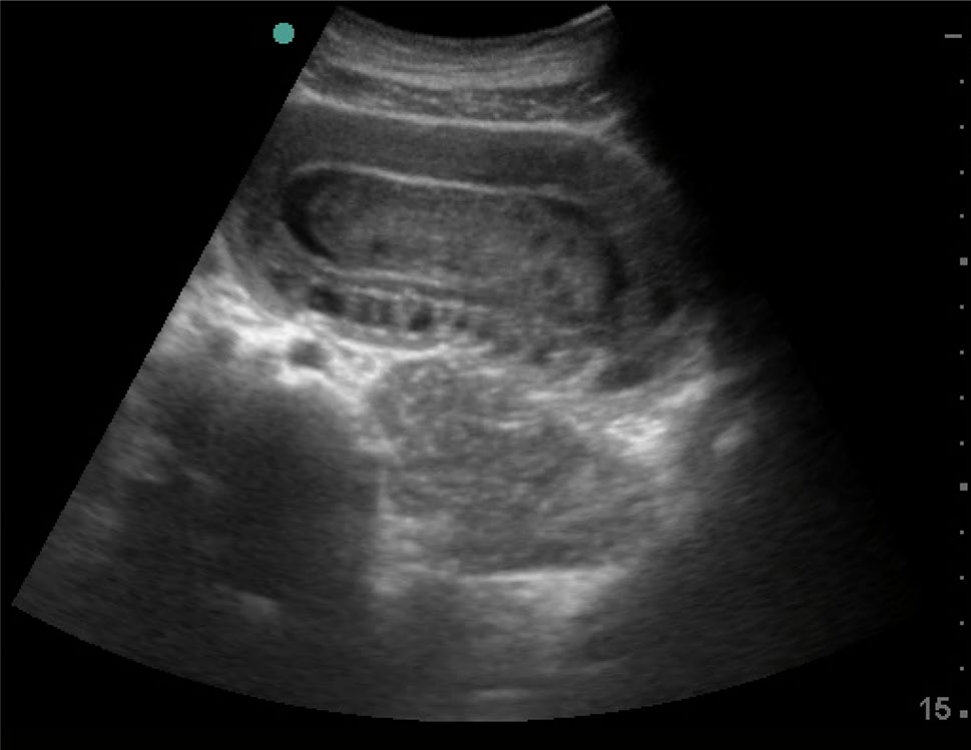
Point of care ultrasound for acute abdomen (ACUTE approach) showed bowel telescoping inside itself with edematous bowel loop wall (long axis)
